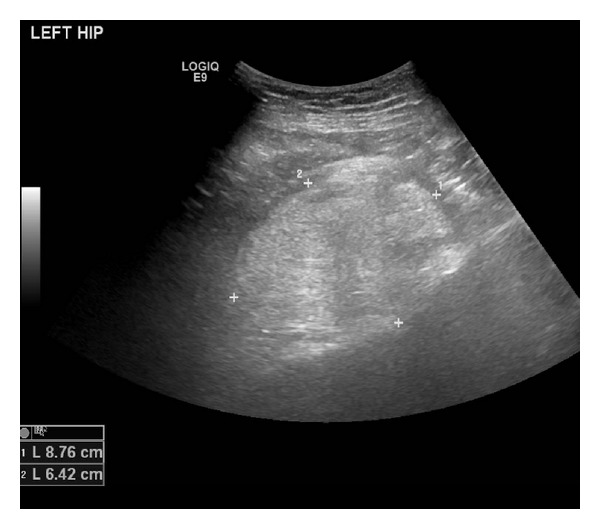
An ultrasound scan demonstrates a hyperechoic lesion, outlined by calliper markings. The homogenous echoic appearance is that of a fat containing lesion.
